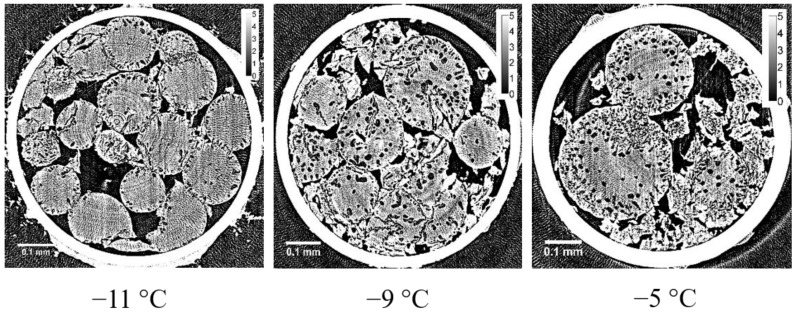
Dependence of X-ray CT images of refrozen G25 beads on the temperature from which the re-cooling was initiated: (left) −11 °C; (middle) −9 °C; (right) −5 °C.
